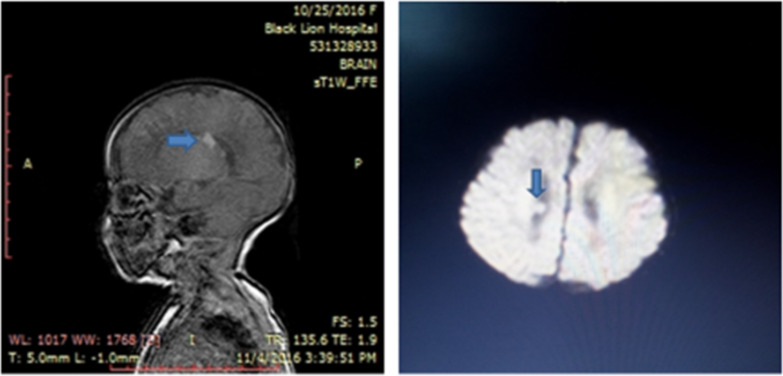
Brain magnetic resonance imaging (MRI) showing subependymal nodules and brain surface tubers
